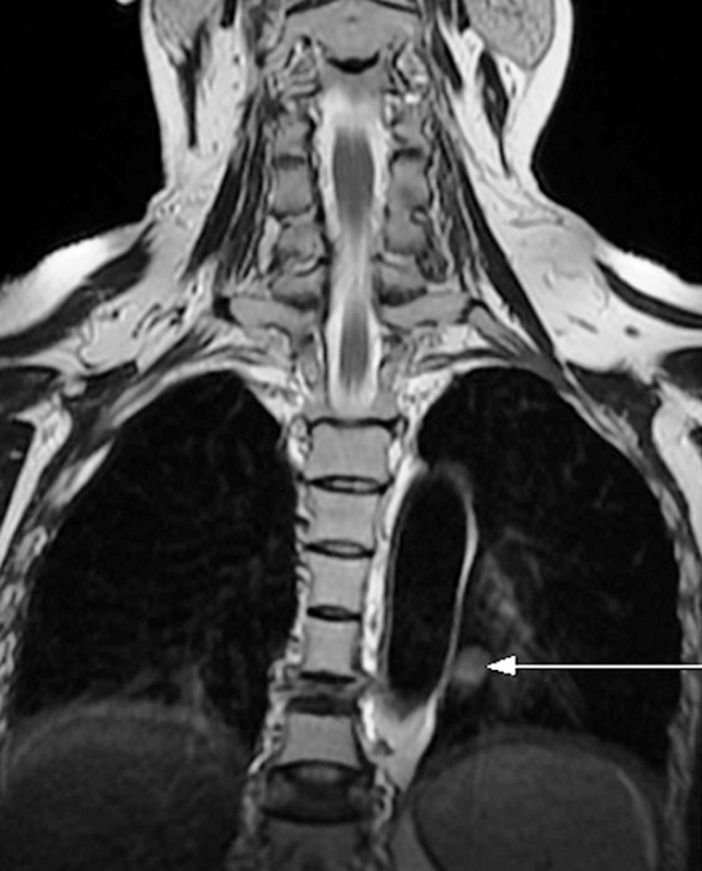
coronal chest MRI T2-weighted revealed one nodular defined lesion in the left lung (arrow)
